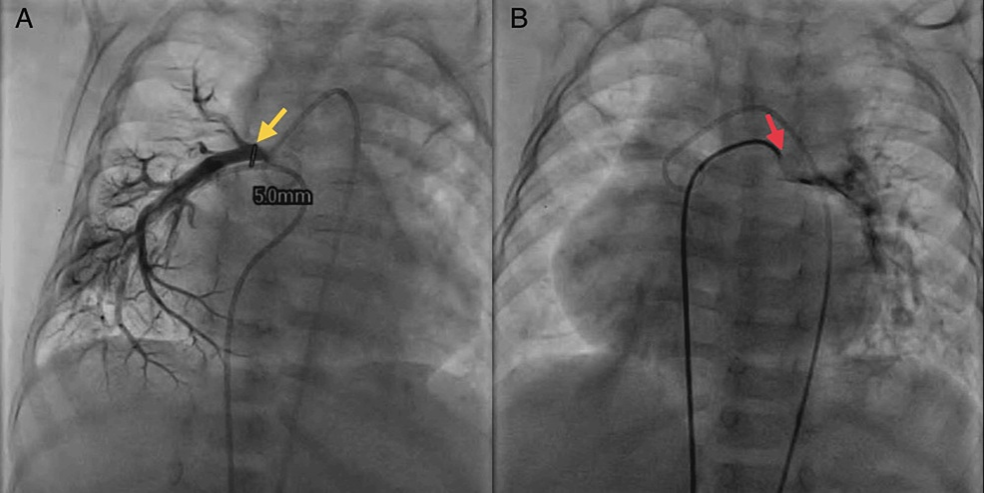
CT angiogram showing (A) LPA measuring 5 mm in size and (B) catheter inserted through IVC to RA to RV to LV to aorta to LPA The image was taken by the authors. IVC: inferior vena cava, RA: right atrium, RV: right ventricle, LV: left ventricle, LPA: left pulmonary artery
